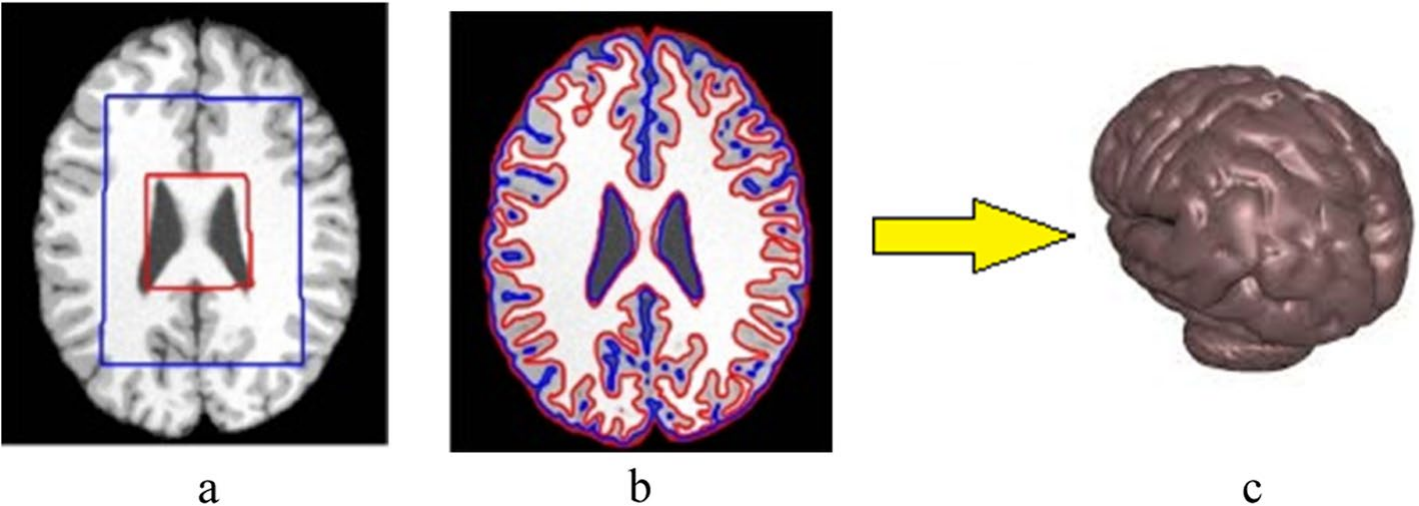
a Brain CT; b Segmented white matter and gray matter; c Reconstructed model
